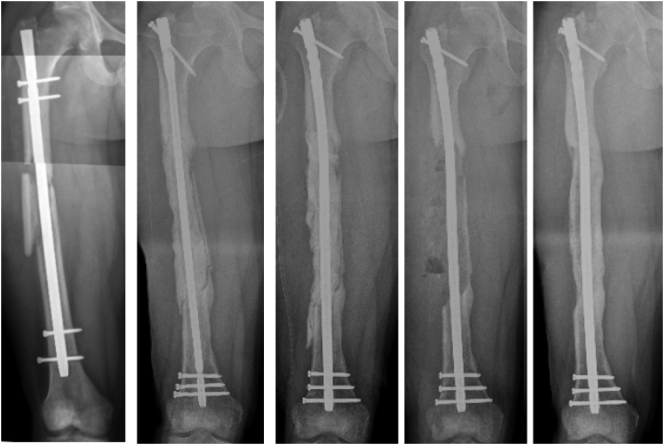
Radiographs demonstrating the patient's progression to complete union of the bone defect.
